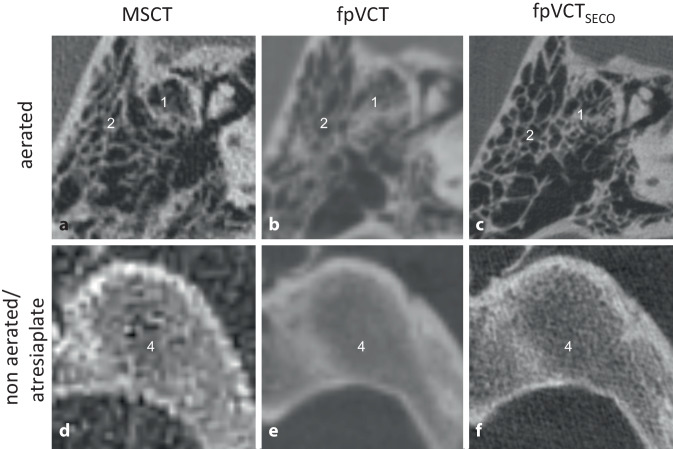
Visualization of the aeration of the mastoid cells. A representative image with a MSCT (600 µm slice thickness), b fpVCT (466 µm slice thickness), and c fpVCT_SECO_ (99 µm slice thickness). d–f Images displaying a non-aerated/atresized plate. *1* Ventilated mastoid cells, *2* bony atresia of the external auditory canal, *4* mastoid atresia plate. *MSCT* multislice computed tomography, *fpVCT* flat-panel volume computed tomography, *fpVCT*_*SECO*_ secondary reconstructions of fpVCT
